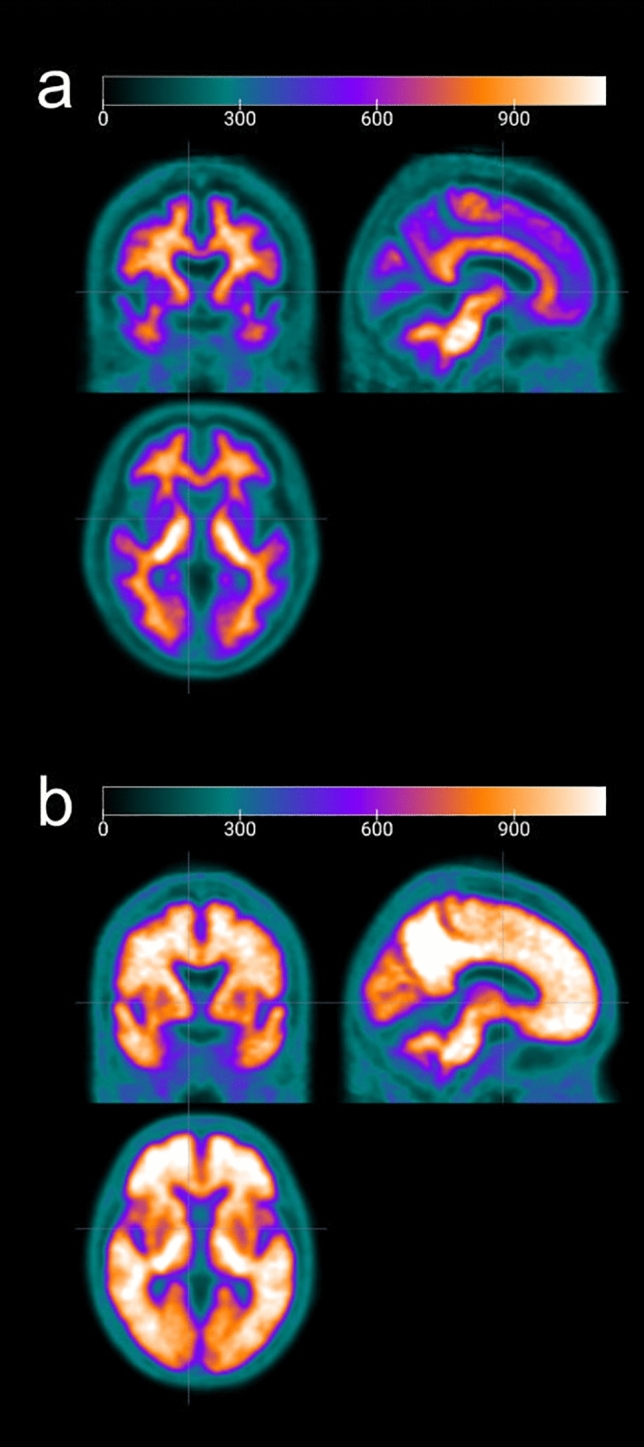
Arithmetic mean-based negative (a) and positive (b) standard brain templates. Each five PET images with the lowest and the highest value of SUVr in ^18^F-flutemetamol accumulation between cerebral cortex and pons were standardized anatomically by using DARTEL, then normalized based on the mean pixel in the pons. DARTEL: Diffeomorphic Anatomical Registration Through Exponentiated Lie Algebra; SUVr: standardized uptake value ratio.
